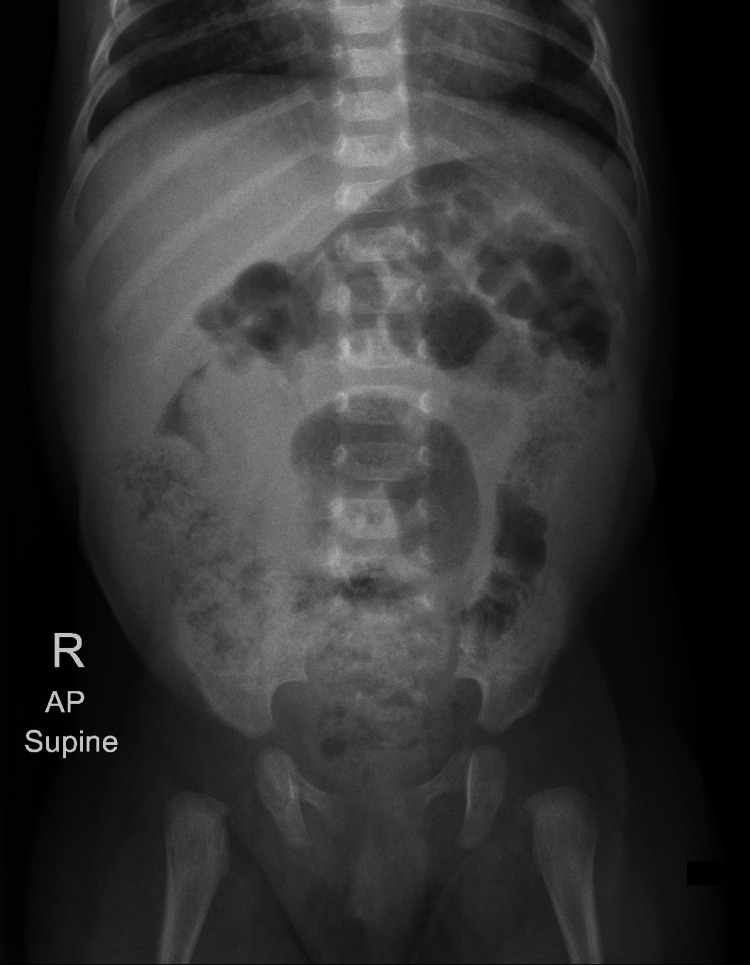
Abdominal X-ray shows a dilated central tubular bowel loop. The colon is loaded with fecal matter.
